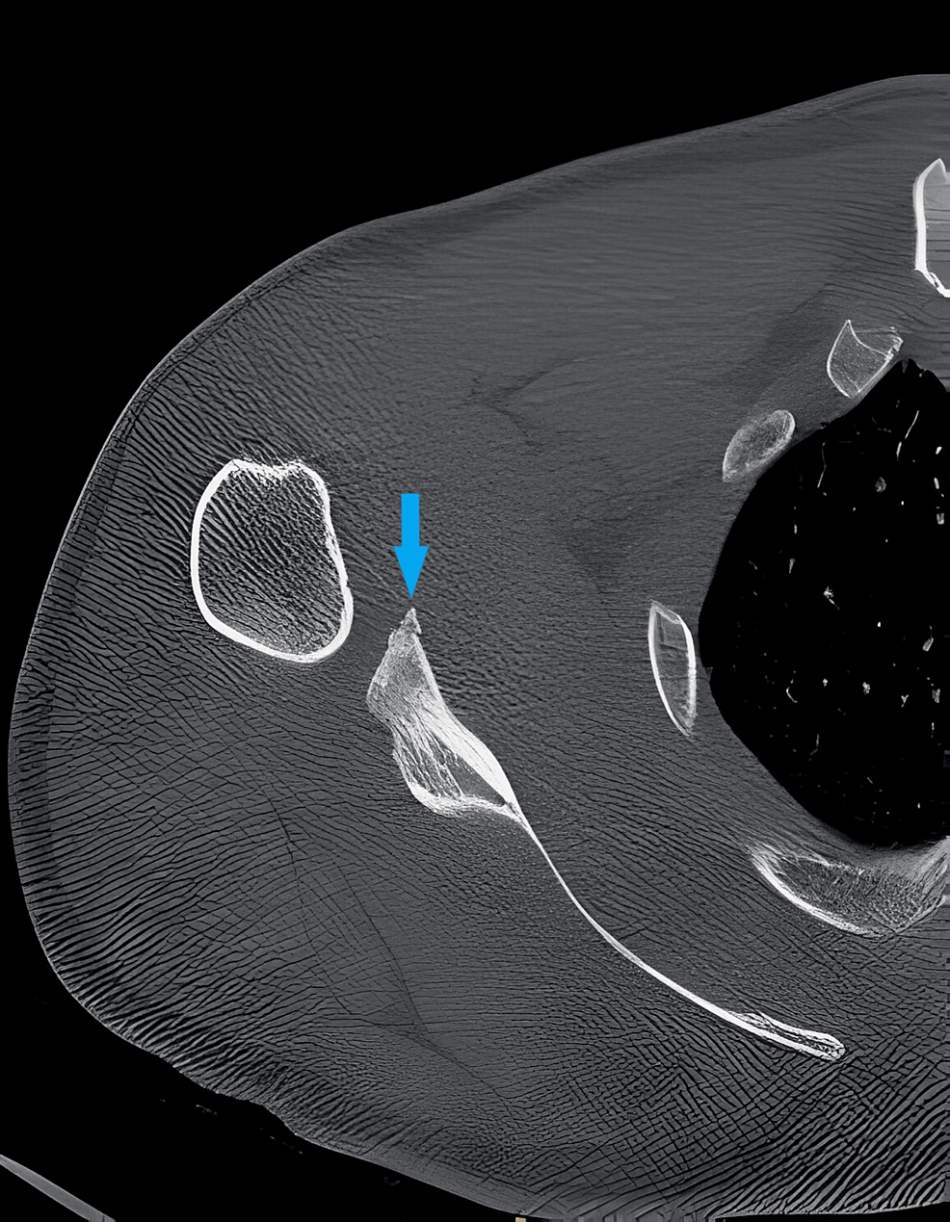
CT scan axial view of right shoulder showing bony Bankart lesion (blue arrow).
